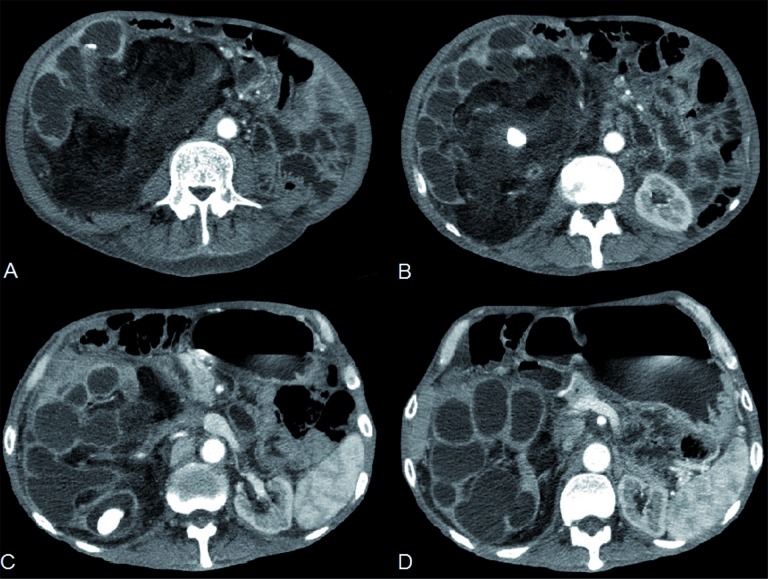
Axial post-contrast CT images show obstructive stones, dilated calyces and renal parenchymal atrophy on the right kidney. Exuberant fibrofatty proliferation in renal sinus, indicating renal replacement lipomatosis (A, B and C). calyceal dilatation with a multiloculated aspect similar to the toe pads of a bear's paw in XGP (D).
